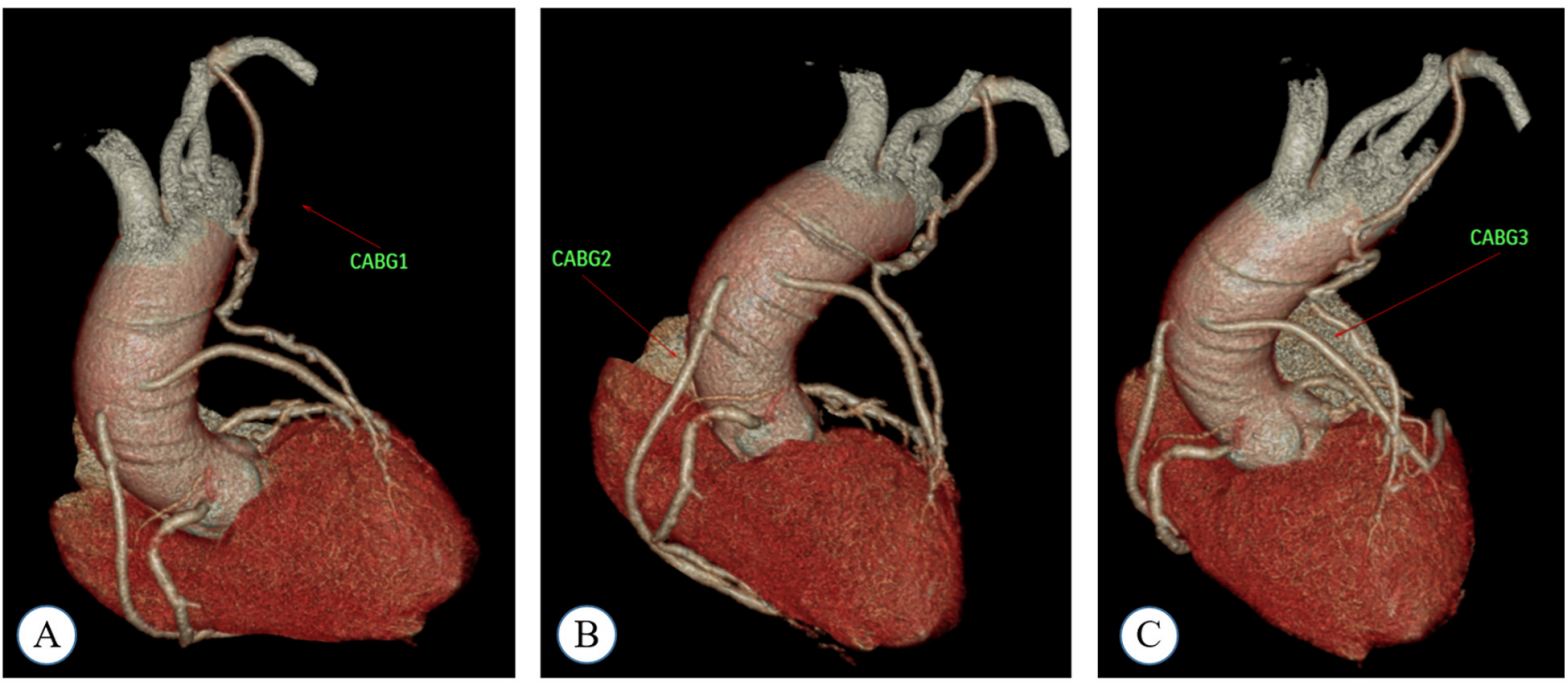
A coronary computed tomography angiography (CTA) image obtained during the postoperative follow-up of a patient who underwent coronary artery bypass grafting (CABG). In this case, the patient received a total of four grafts: LIMA-LAD, AO-SVG-PDA, and AO-SVG-DIG-OBM. Specifically, the LAD artery was targeted for CABG 1, and a LIMA graft was employed as the conduit. For CABG 2, the PDA was the target vessel, and an SVG graft was utilized. CABG 3 involved the target vessels DIG and OBM, with an SVG graft used for the bypass. (A) shows CABG1, (B) shows CABG2, and (C) shows CABG3.
